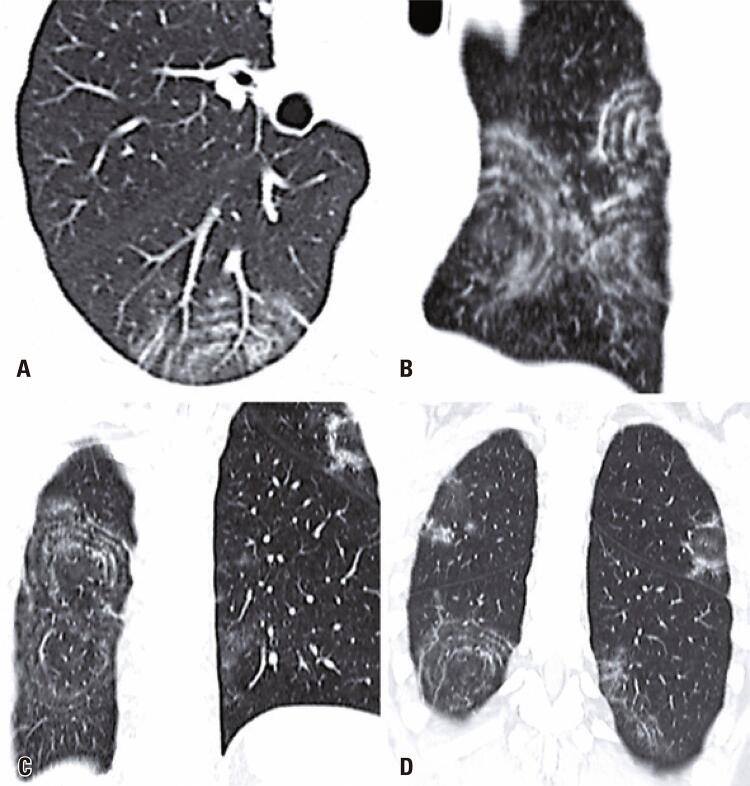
Chest computed tomography imaging (A) axial, (B) sagittal oblique, and (C and D) coronal oblique show the target sign characterized by multiple concentric ring-like opacities, with a central nodular peribronchovascular opacity, in a 49-year-old man with COVID-19 pneumonia. Note the reversed halo sign in the left upper lobe
